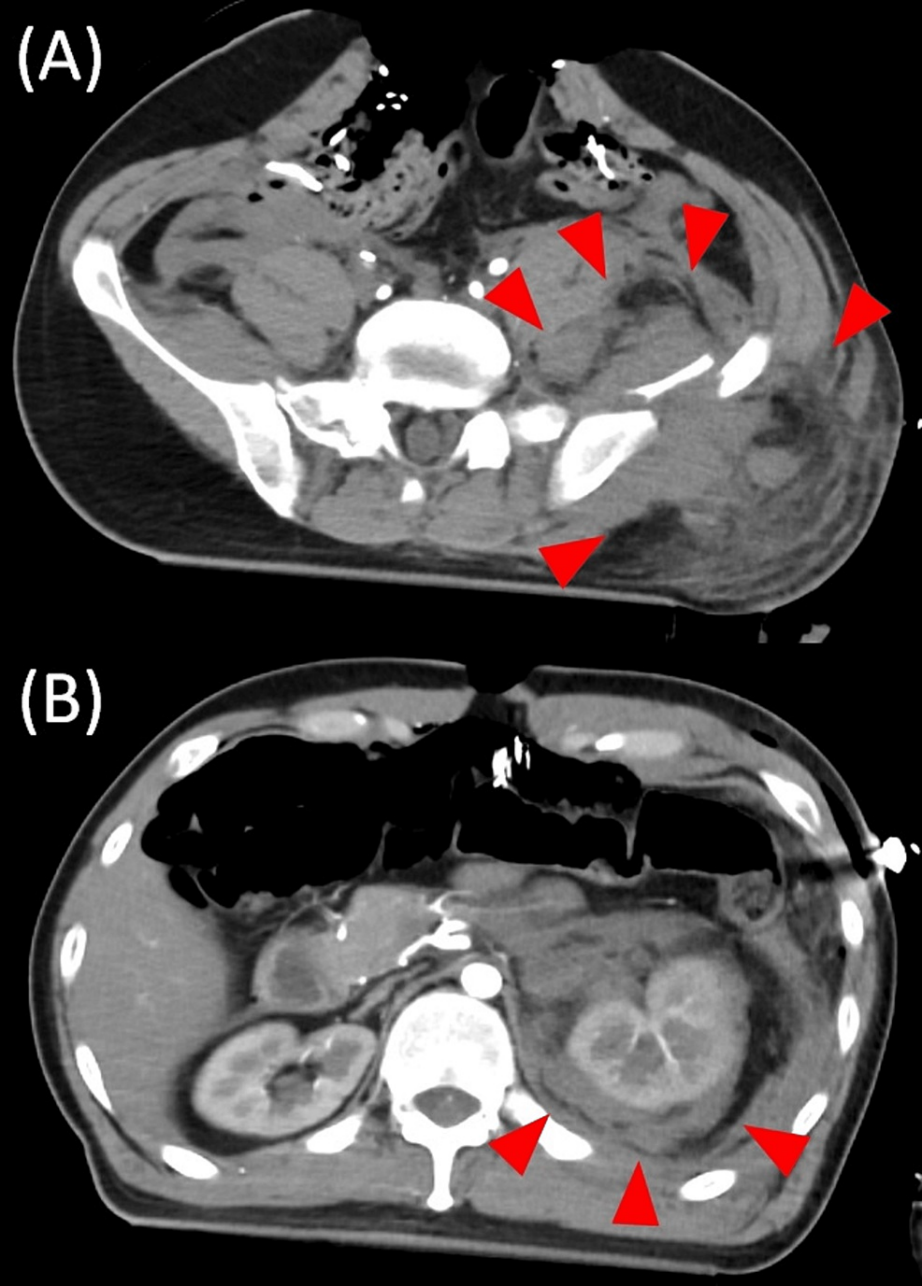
CT of the pelvis and abdomen. (A) CT showed obvious hematoma (arrowheads) from around the pelvic fracture to (B) the perirenal space.
